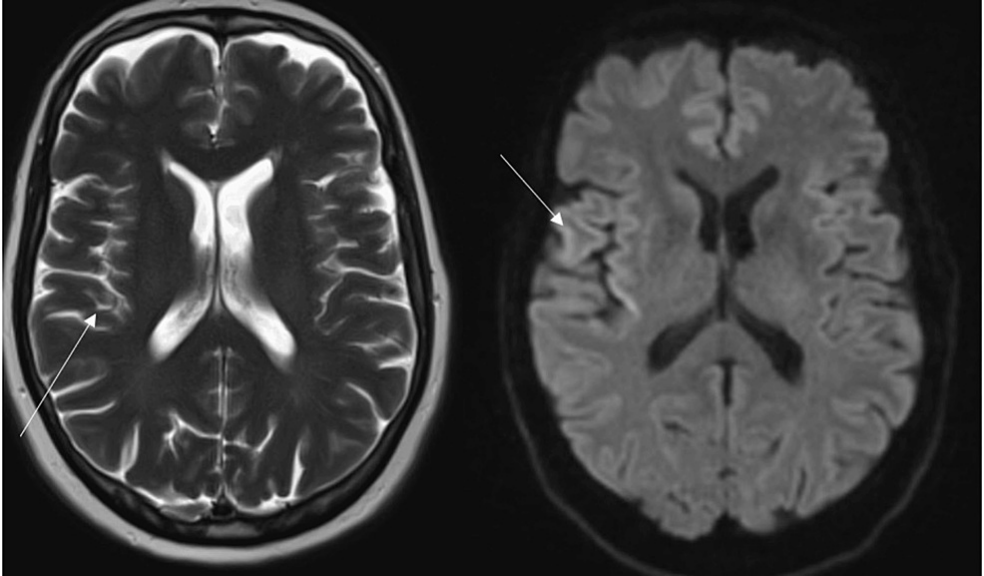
MRI showing hyper-intensity along the frontal and temporo-parieto-occipital region. White arrows indicate hyper-intensity
